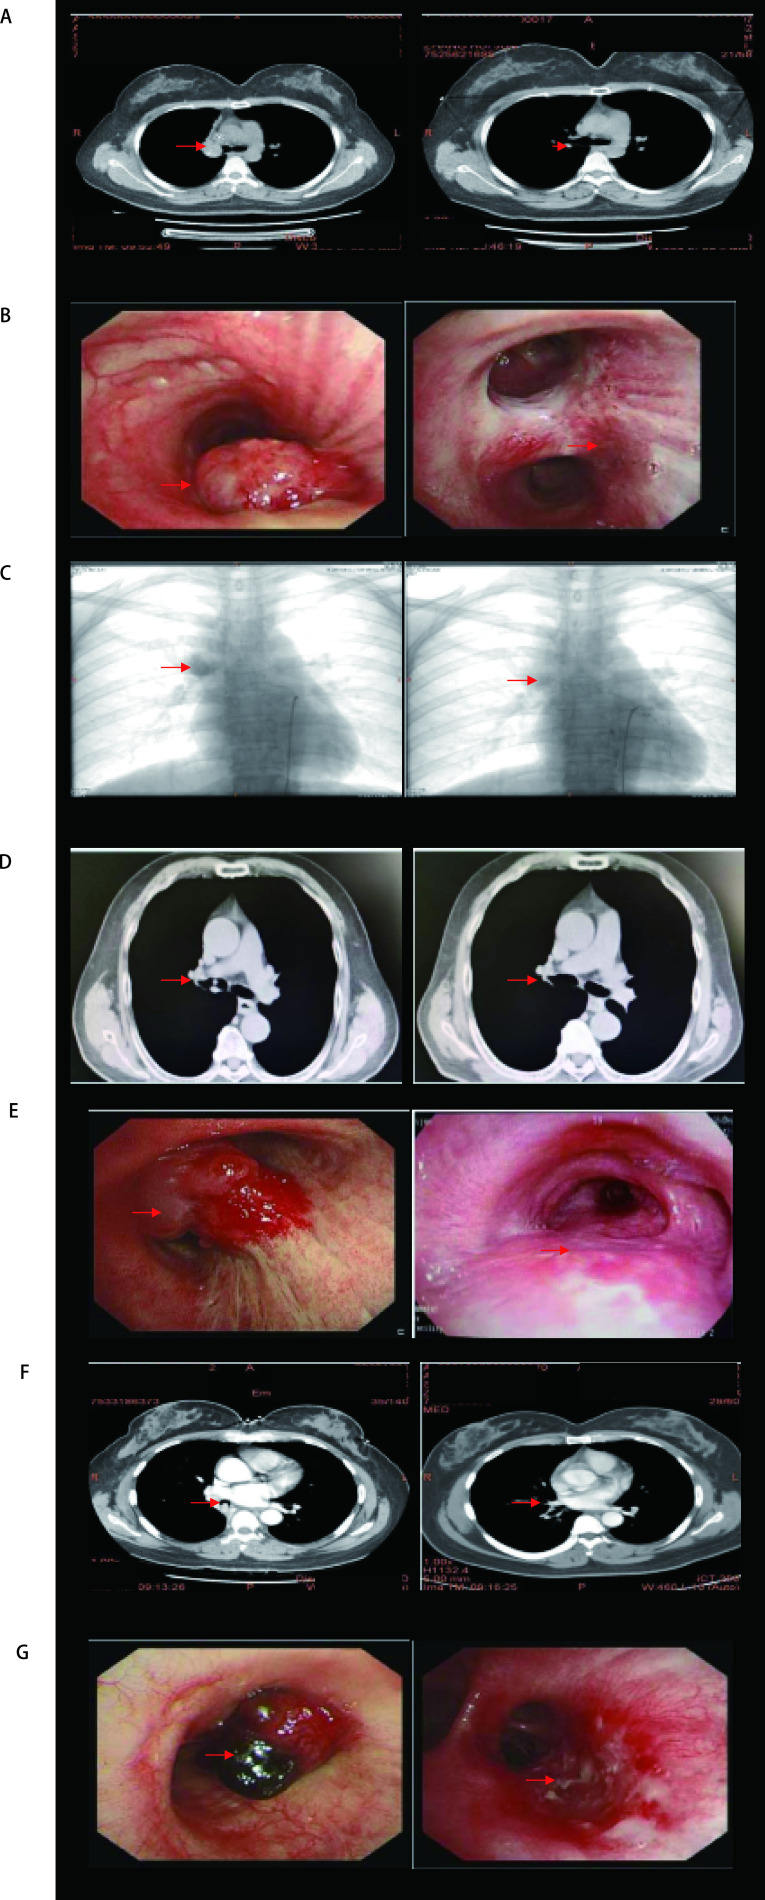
（A、B、C）对比图：A：病例13支气管镜介入治疗前后胸部CT对比；B：病例13支气管镜介入治疗前后对比；C：病例13支气管介入栓塞术前后对比；（D、E）对比图：D：病例14支气管镜介入治疗前后胸部CT对比；E：病例14支气管镜介入治疗前后对比；（F、G）对比图：F：病例16支气管镜介入治疗前后胸部CT对比；G：病例16支气管镜介入治疗。 Comparison chart (A, B, C). A: Comparison of thoracic CT scan in the before and after the bronchoscopic treatment for case 13; B: Comparison of bronchoscopy in the before and after the bronchoscopic treatment for case 13; C: Comparison of image outcome in the before and after the chemotherapy with bronchial arterial embolization for case 13. Comparison chart (D, E): D: Compari-son of thoracic CT scan in the before and after the broncho-scopic treatment for case 14; E: Comparison of bronchoscopy in the before and after the bronchoscopic treatment for case 14. Comparison chart (F, G): F: Comparison of thoracic CT scan in the before and after the bronchoscopic treatment for case 16; G: Comparison of bronchoscopy in the before and after the bronchoscopic treatment for case 16. CT: computed tomography.
